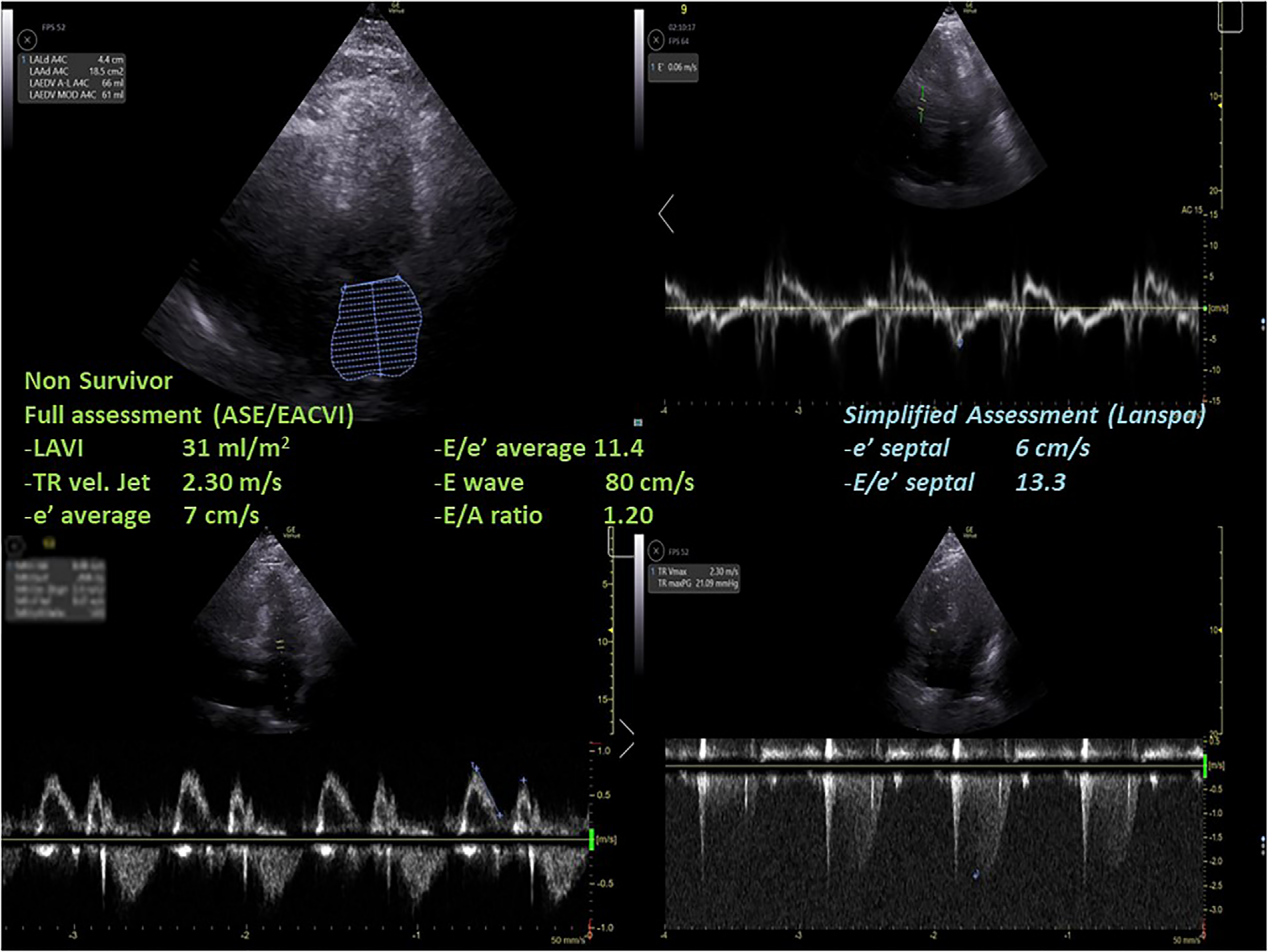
A case of a non‐survivor where the assessment with full criteria resulted in a normal left ventricular diastolic function, while using the simplified assessment according to Lanspa criteria a diagnosis of grade III left ventricular diastolic dysfunction was made. ASE, American Society of Echocardiography; EACVI, European Association of Cardiovascular Imaging; LAVI, left atrial volume index; TR, tricuspid regurgitation.
